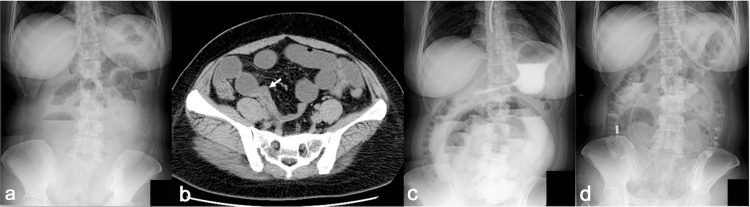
(A) Abdominal X-ray image (standing position) on day 0. (B) Plain CT image at the site of caliber change (arrow). (C) Abdominal X-ray image (standing position) three hours after WSC injection through the nasogastric tube on day 3, showing multiple air-fluid levels. (D) Abdominal X-ray image (standing position) two days after long intestinal tube placement. CT, computed tomography; WSC, water-soluble contrast
